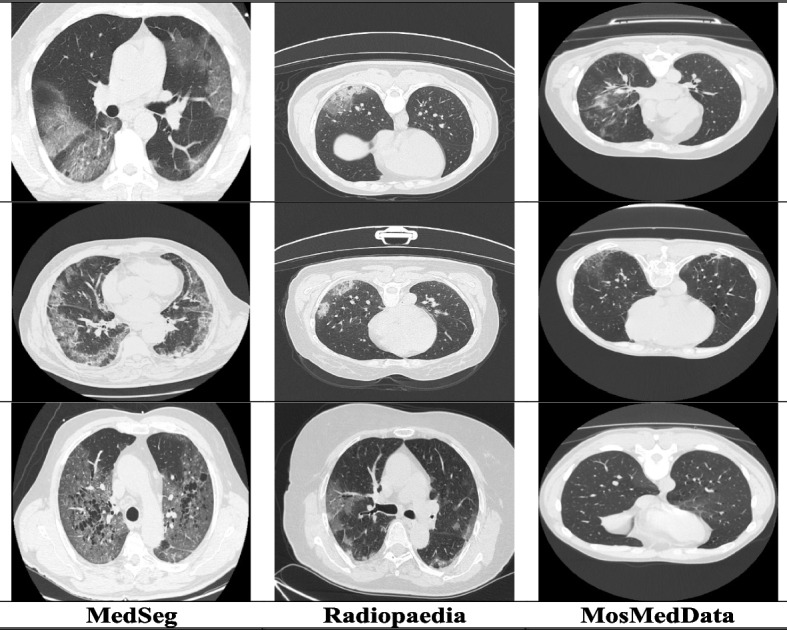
Visualization of the heterogeneity of COVID-19 CT samples orginating from different sites
